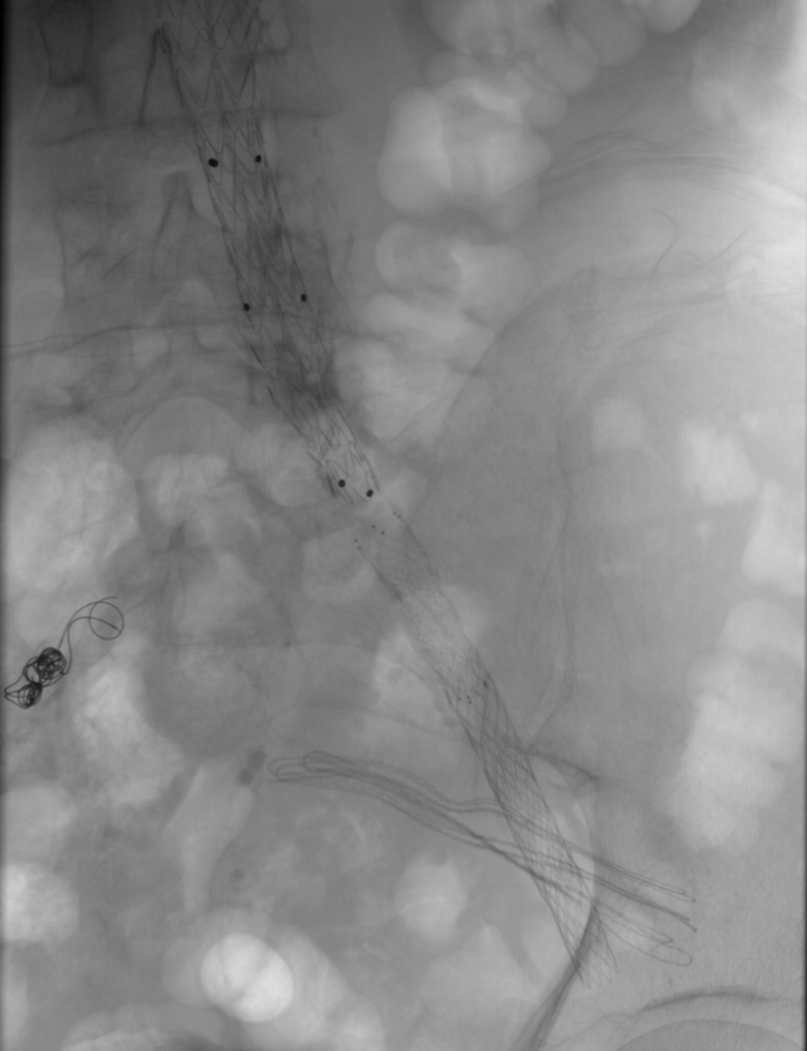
X-ray of the completed reconstruction with stent graft, leg extension, and microcoils.
